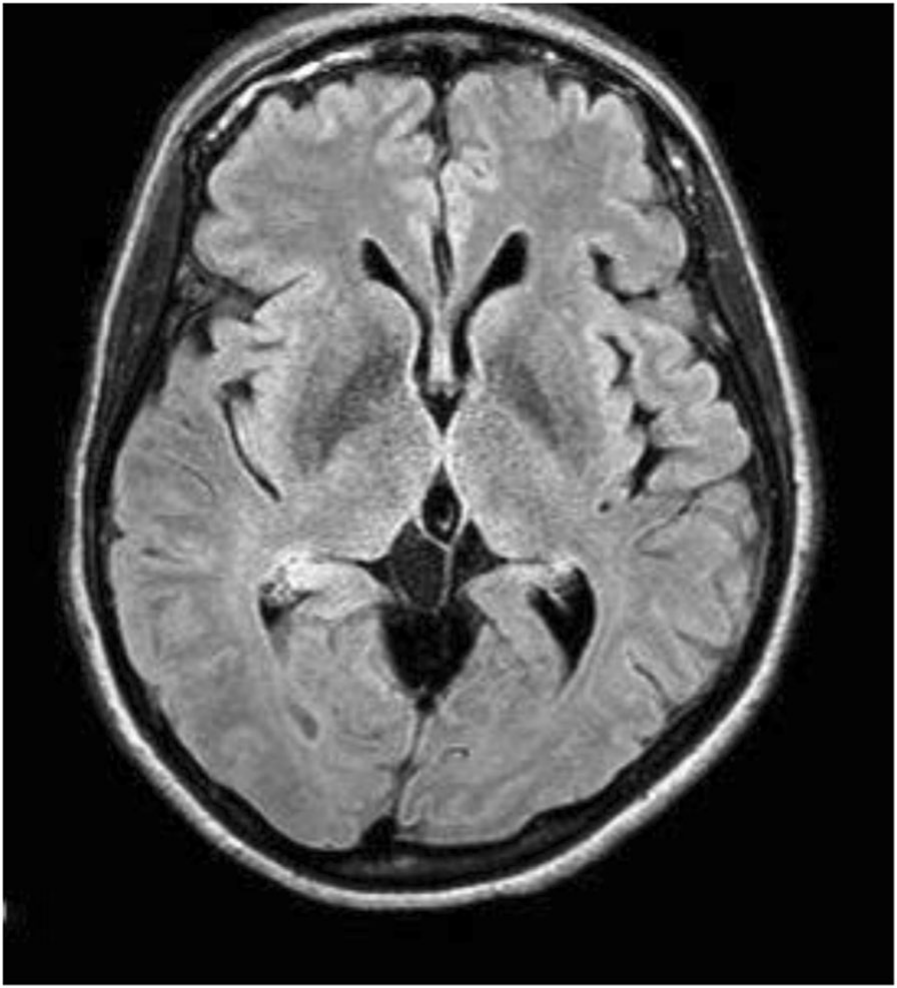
Magnetic resonance of head with T2-weighted-Fluid-Attenuated Inversion Recovery with intravenous gadolinium showing debris in the right lateral ventricle and dural enhancement consistent with meningitis
